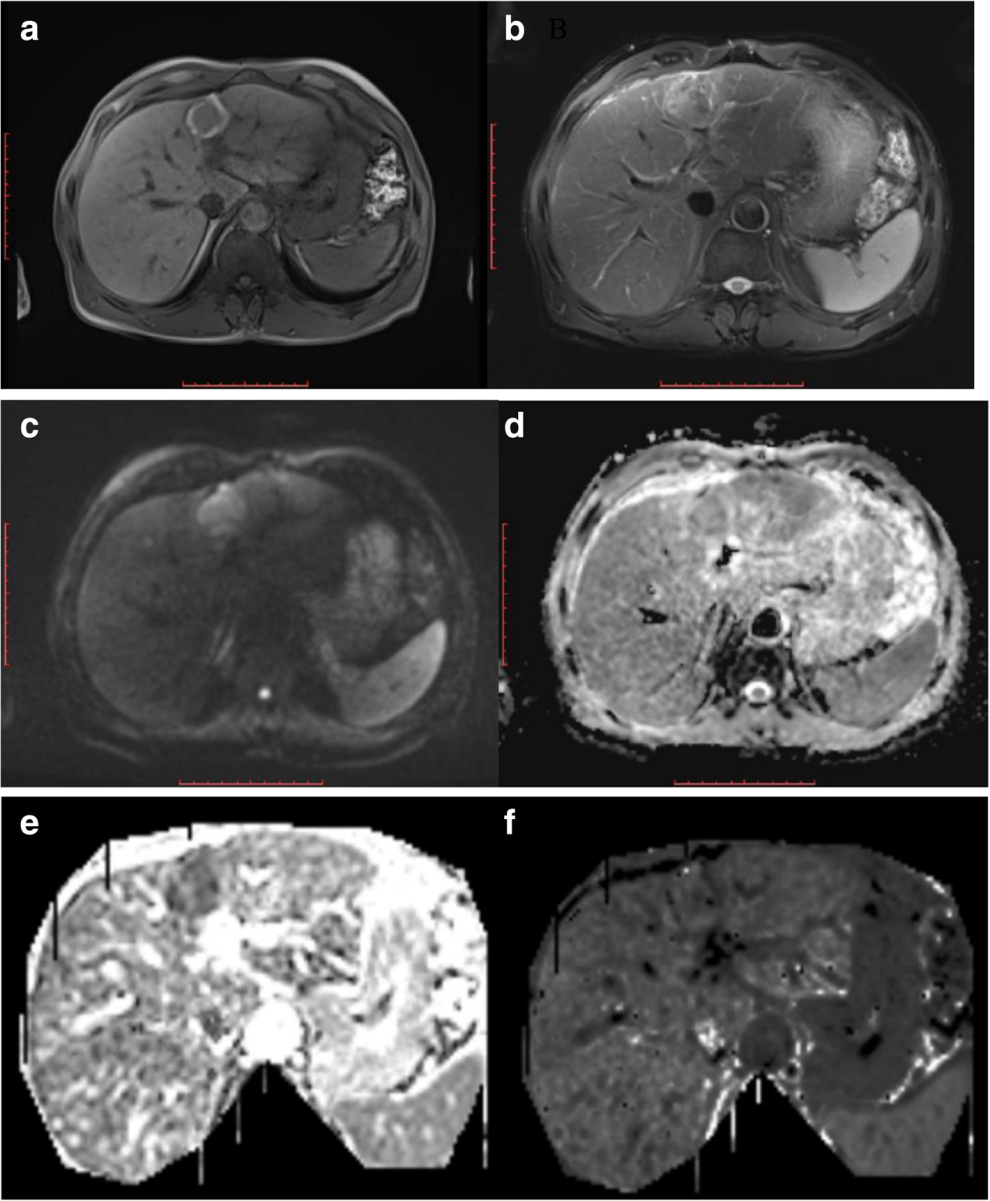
(The same patient in Fig. 2, after treatment by RFA for one month) a axial T1WI and b axial fat-saturated T2WI indicate that the signal strength of the lesion is reduced compared with that before. c and d: axial DWI and ADC map show that the diffused degree of the lesion is obviously reduced. e DKI-MD map show that the MD value for the lesion is 1.300 × 10^− 3^. f DKI-MK map show that the MK value for the lesion is 5.906 × 10^− 1^
